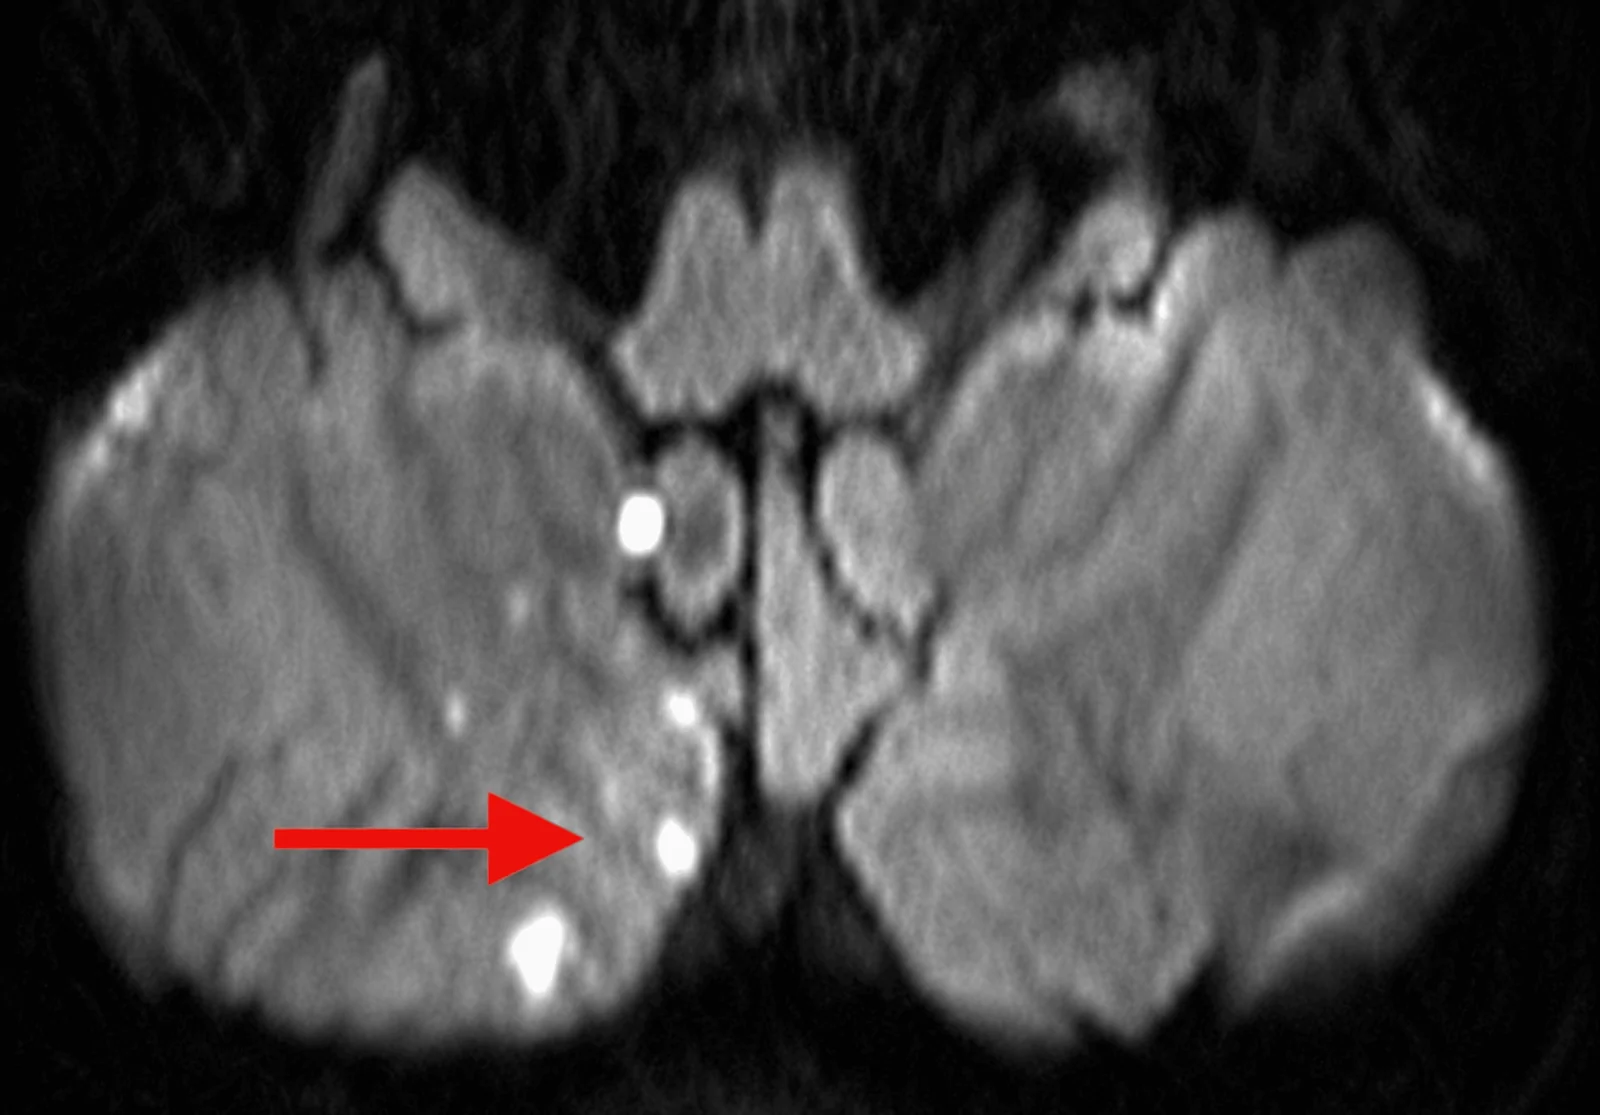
Diffusion-weighted magnetic resonance imaging demonstrating right cerebellar infarcts Axial diffusion-weighted magnetic resonance imaging demonstrates multiple hyperintense foci in the inferomedial right cerebellar hemisphere, consistent with acute-to-subacute infarcts. The red arrow indicates a representative focus. A separate acute-to-subacute infarct in the posterior right medulla was identified on another image from the examination and is not shown in this figure
